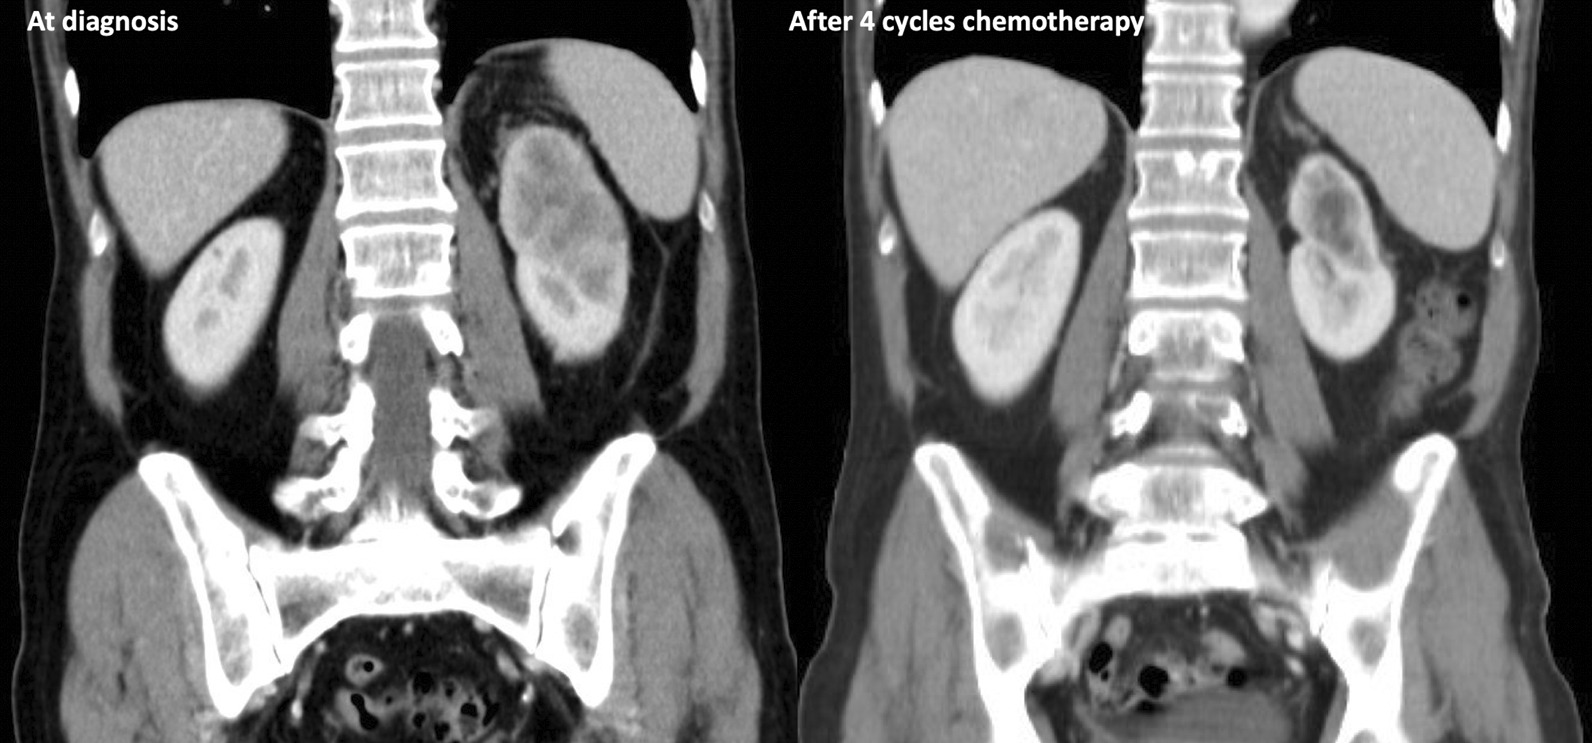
Pretreatment computed tomography scan with the presence of a large collecting duct carcinoma of the left kidney at diagnosis (left). Note the decrease in the size of the primary tumor after four cycles with gemcitabine/cisplatin chemotherapy (right)
